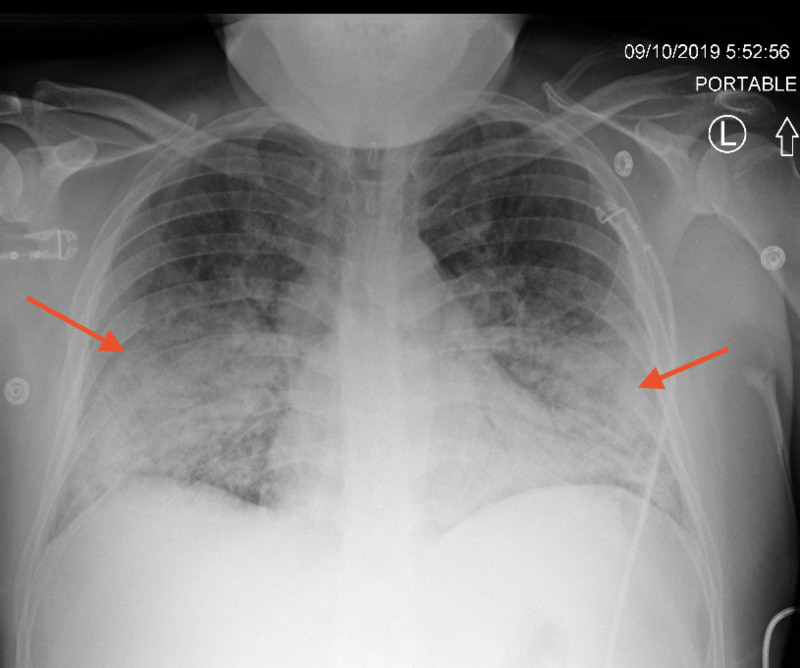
Chest x-ray on Day 3 of admission showed rapidly worsening bilateral lower lobe infiltrates with subpleural sparing (red arrows).
